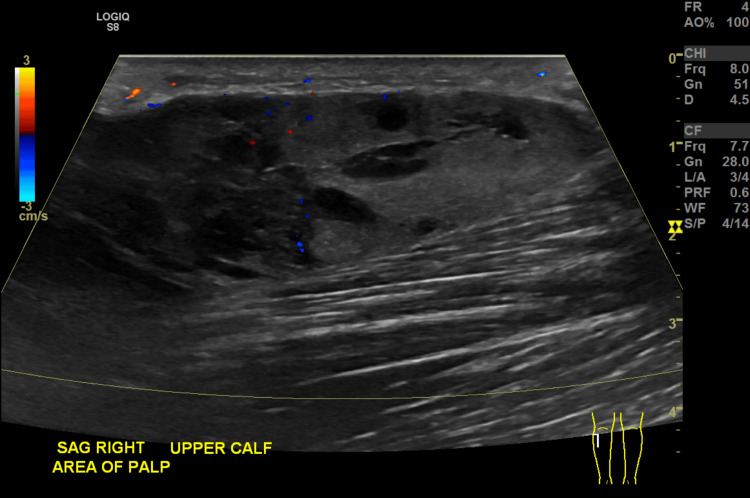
Right lower extremity ultrasound with color Initial imaging of a 9.1 × 2.2 × 4.4 cm heterogeneous, mostly hypoechoic mass without significant internal vascular flow overlying the imaged calf muscles, suggestive of hematoma.
